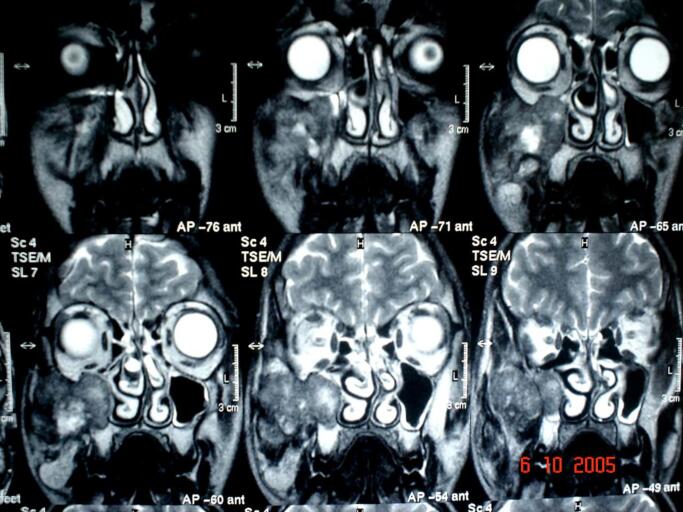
MRI (coronal view) showing invasive lesion which invades the sinus maxillaris and penetrates to the orbital floor and zygomatic bone.
